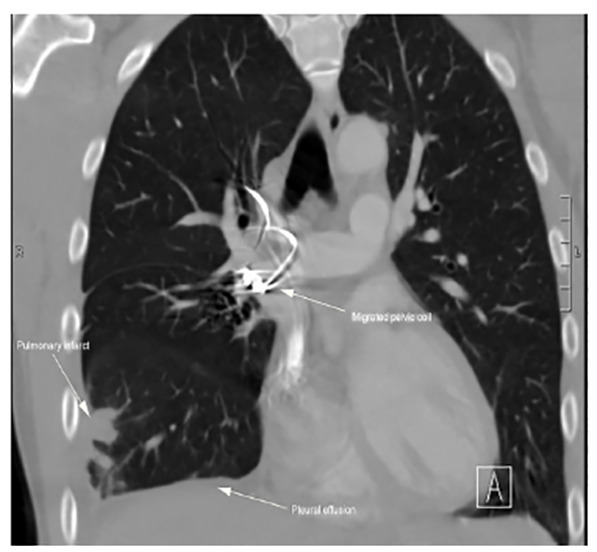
Computed tomography imaging showing a migrated pelvic coil (mid-image arrow) in the right pulmonary artery with areas of pulmonary infarct in the right middle and lower lobes and a small right pleural effusion (left and lower arrows).
